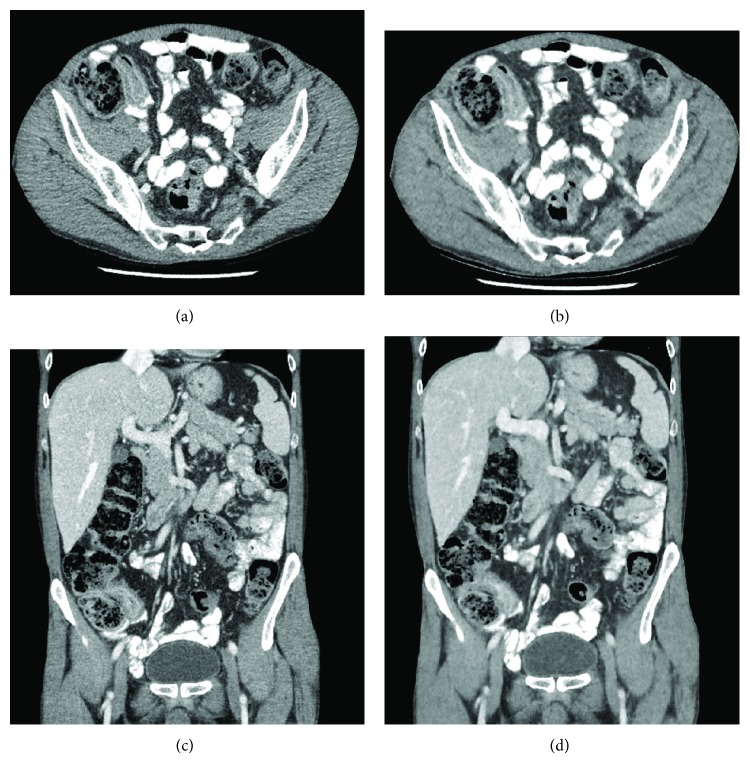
(a, c) Conventional dose axial and coronal CT reconstructed with ASIR and (b, d) low-dose axial and coronal CT reconstructed with MBIR demonstrating mural thickening at the terminal ileum and adjacent mild fat stranding consistent with acute inflammation. The low-dose CT entails an approximately 75% radiation dose reduction.
